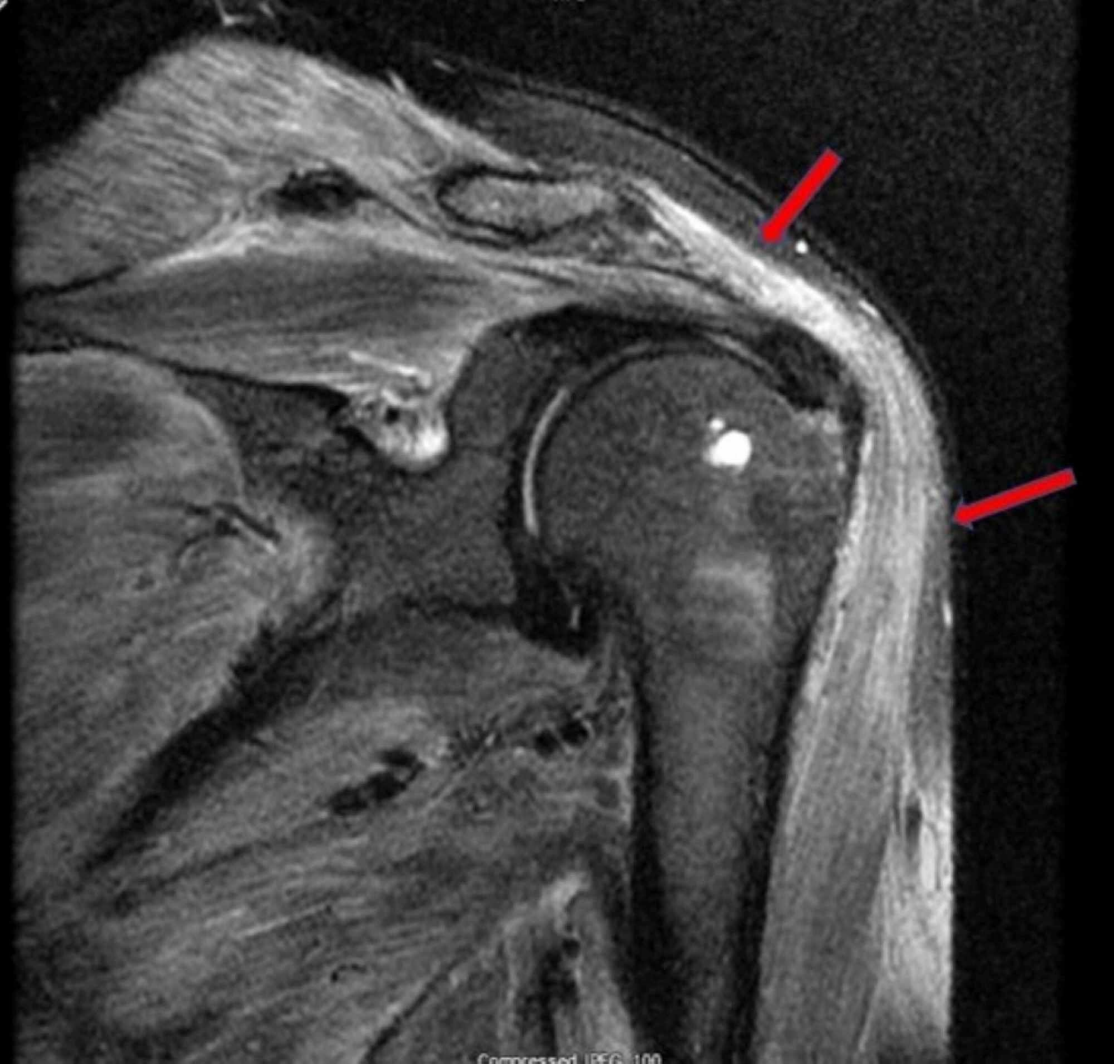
MRI of the left shoulder without contrast The image shows diffuse muscle edema on T2-weighted image (arrows) MRI: magnetic resonance imaging
